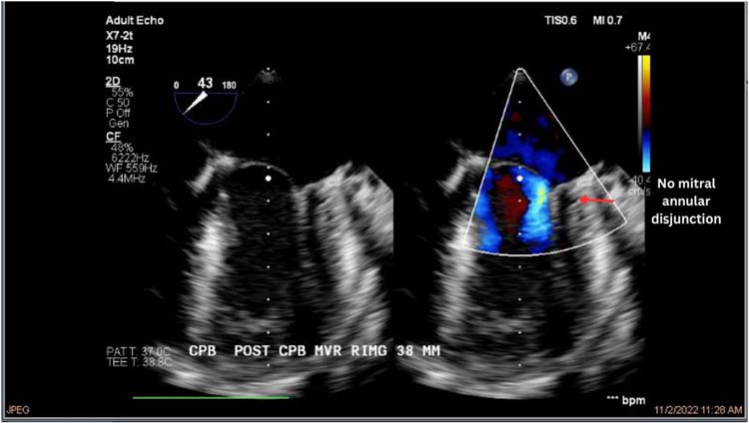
Postoperative echocardiography of patient 2.
